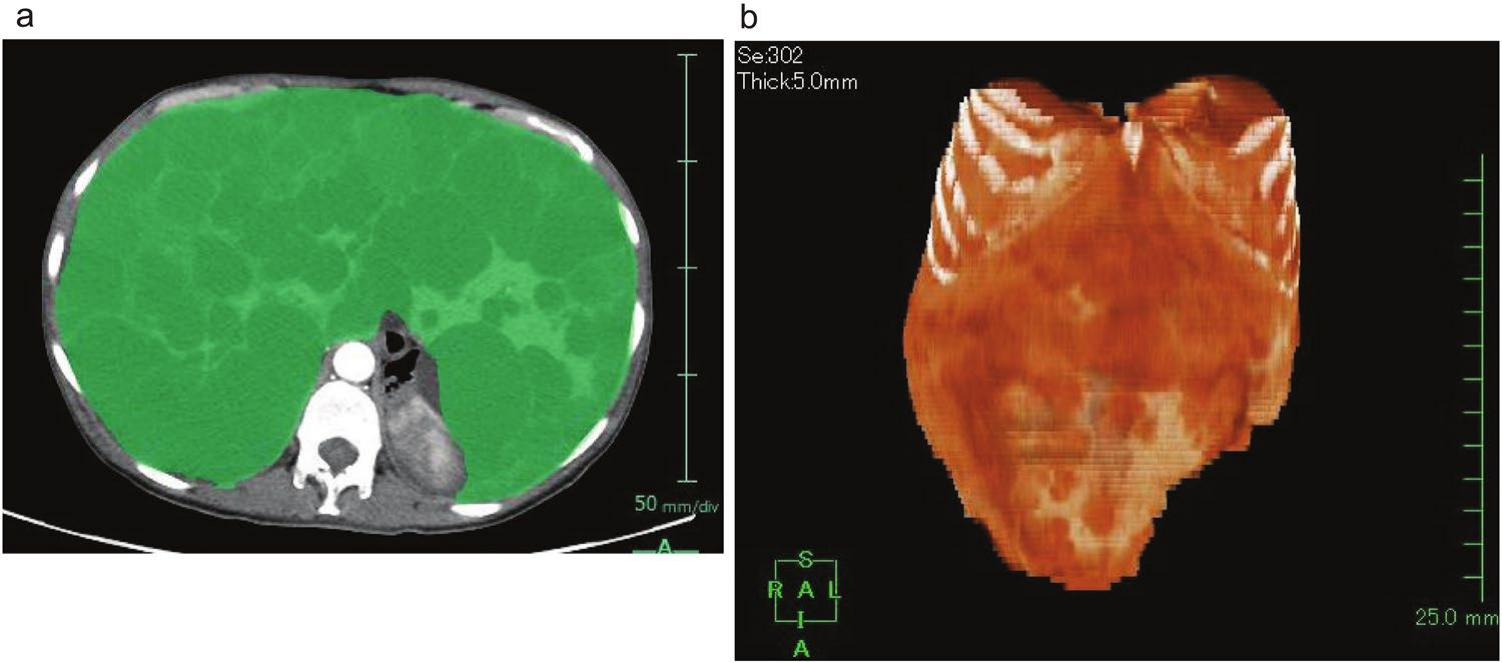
Measurement of liver volume using an image workstation (SYNAPSE VINCENT®, Fujifilm Medical Systems, Tokyo, Japan). The periphery of the liver parenchyma is manually extracted in each CT image slice in the coronal section (3–5 mm thickness) a The workstation creates a 3D structure of the whole liver parenchyma, and the liver volume is automatically measured from the extracted area (b)
